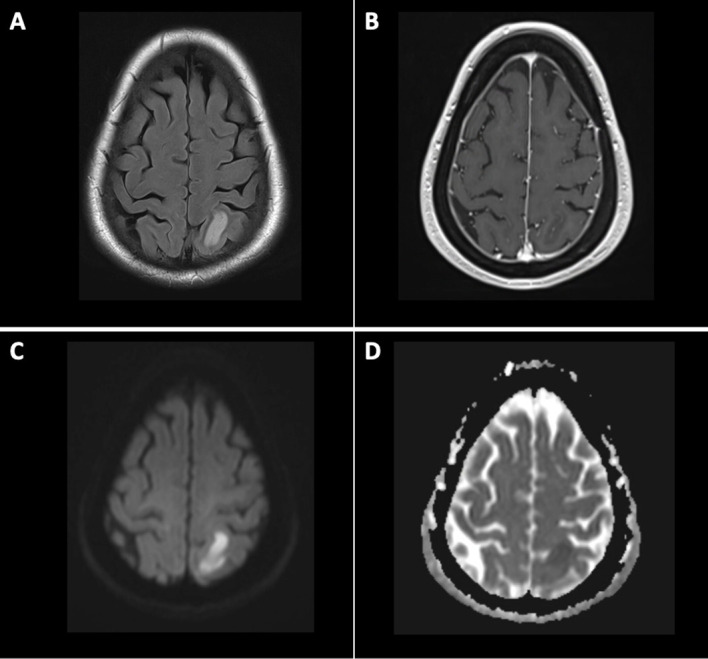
MRI on post CAR-T day +21. MRI Brain with and without contrast showing T2 hyperintensity (A), lack of enhancement on T1 post-contrast (B), diffuse restriction (C), and corresponding ADC correlate (D).
